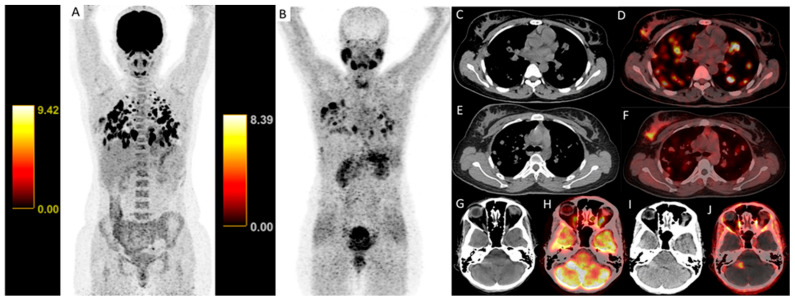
Maximum-intensity projection (MIP) and axial positron emission tomography/computed tomography (PET/CT) images demonstrate differential tracer uptake in a patient with metastatic breast cancer. Panels a and b show whole-body MIP images for [18F]FDG (A) and [68Ga]Ga-DOTA.SA.FAPi (B), highlighting the primary tumor and multiple metastatic lesions, including lung metastases. Axial computed tomography (CT) images and fused PET/CT images ([18F]FDG PET/CT: (C,G), (D,H); [68Ga]Ga-DOTA.SA.FAPi PET/CT: (E,I); (F,J) respectively) are shown. Axial brain CT (I) and fused PET/CT (J) images demonstrate a metastasis in the right cerebellar hemisphere with higher lesion-to-background contrast on [68Ga]Ga-DOTA.SA.FAPi. SUV color bars displayed adjacent to the MIP images indicate the SUV scale used for PET image display and apply to both the MIP and corresponding axial fused images (SUV scale: 0–9.42 for [18F]FDG and 0–8.39 for [68Ga]Ga-DOTA.SA.FAPi). Abbreviations: MIP, maximum-intensity projection; PET, positron emission tomography; CT, computed tomography.
